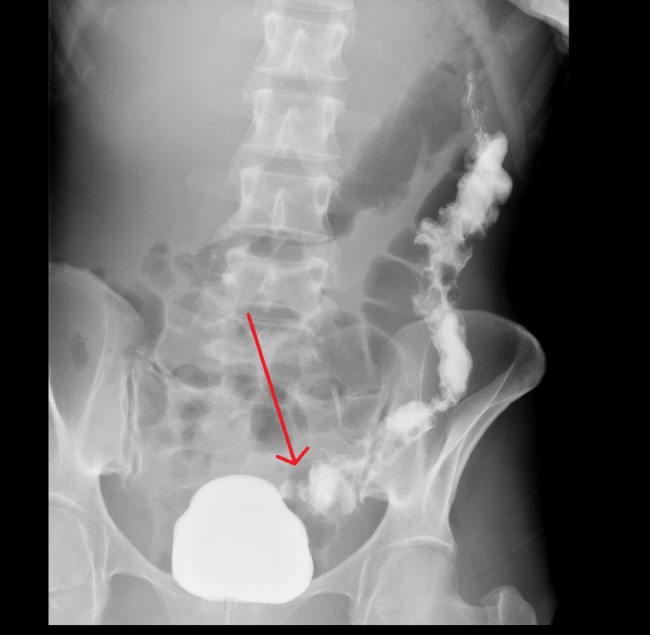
X-ray cystogram showing colovesical fistula (red arrow) connecting the bladder to the proximal sigmoid colon
